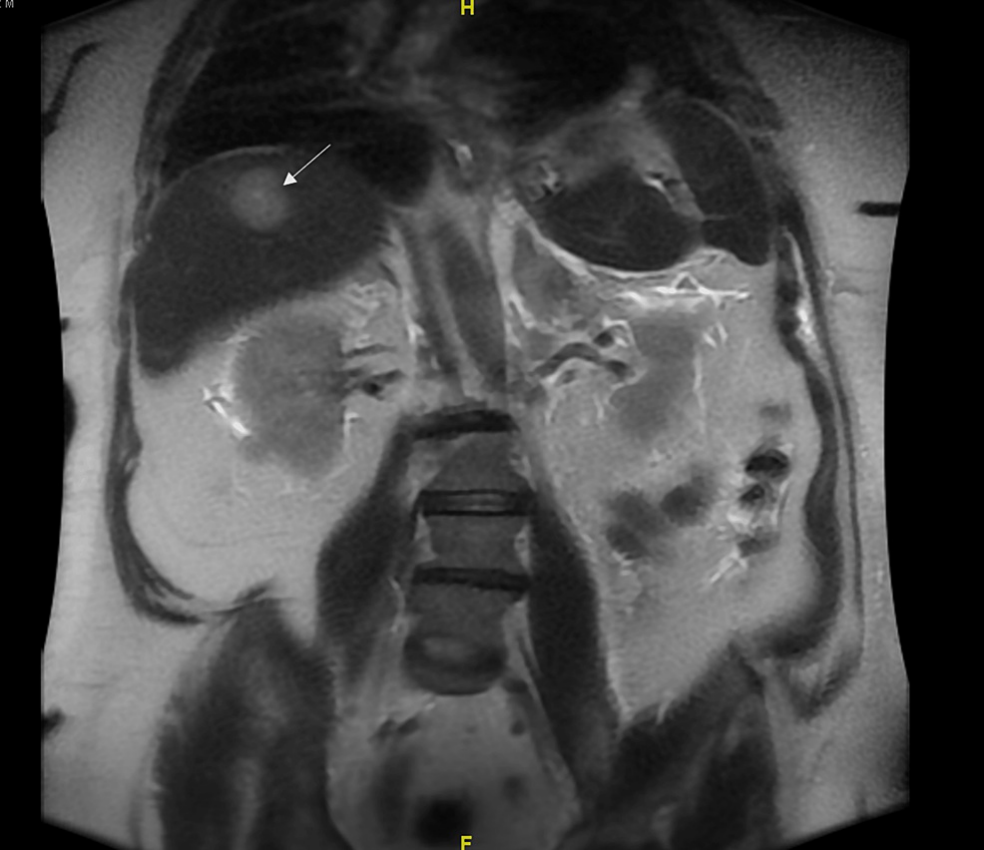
MRI of the abdomen showing a liver lesion measuring up to 3.6x3.2 cm.
